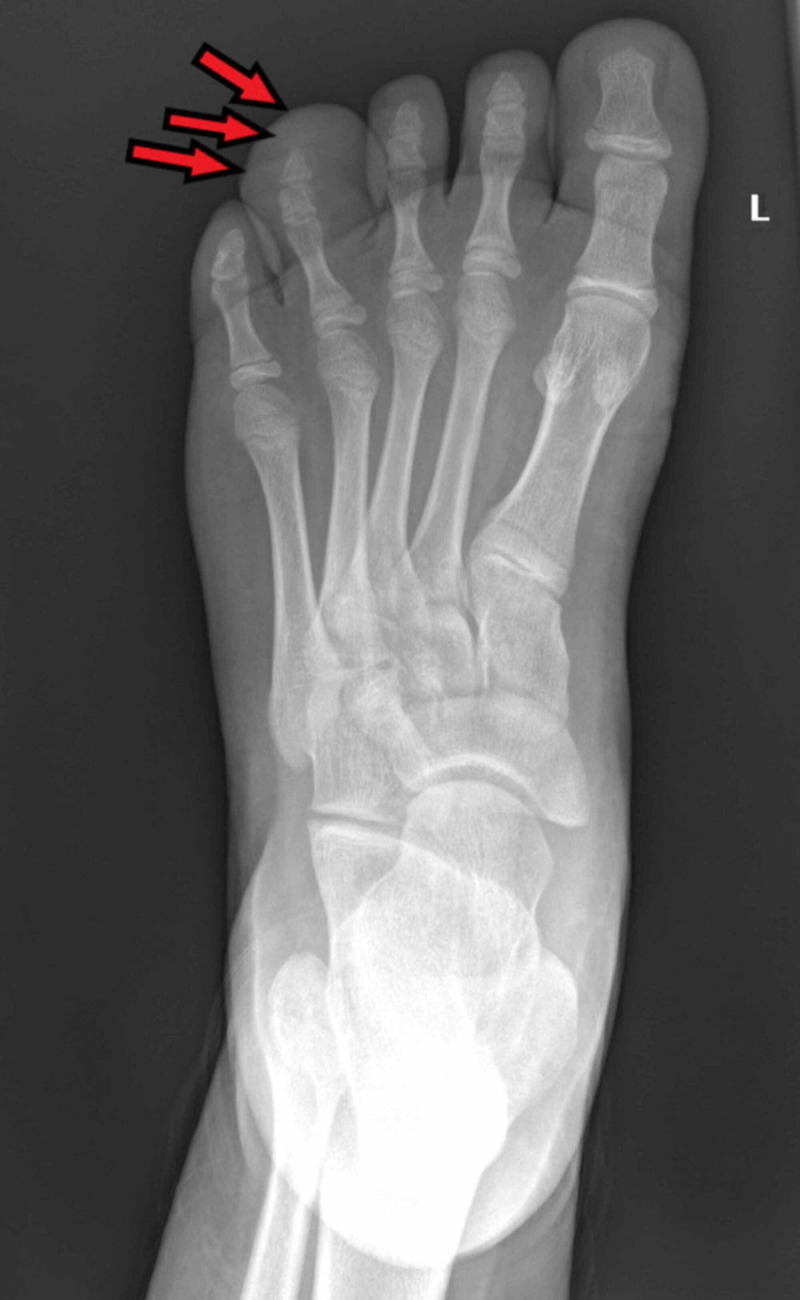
X-ray image of the left foot. Red arrows in the image show the soft enlargement of the affected digit, but the underlying bone is normal with no obvious hypertrophic changes.
